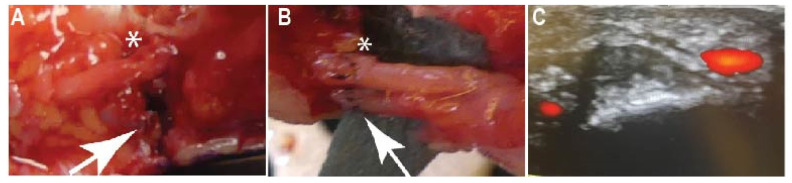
Representative images of the study cohorts. (A) A one-vessel blood supply patient without artery reconstruction (arrow) and with nerve reconstruction (asterisk). (B) A two-vessel blood supply patient with a reconstructed artery (arrow) and nerve (asterisk). (C) Doppler sonography of two intact arteries (red).
